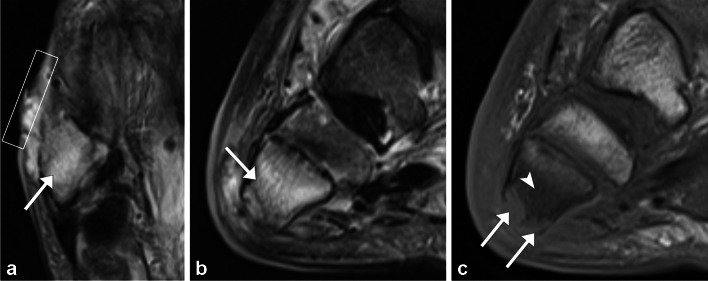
Seventy-one-year-old diabetic man with fifth ray infection status posttransmetatarsal amputation with positive margins. Long (a) and short (b) axis STIR images through the foot obtained several weeks after surgery demonstrate bone marrow edema like signal intensity approaching that of fluid within the fifth metatarsal stump (arrows). There is adjacent skin irregularity and abnormal T2 hyperintense subcutaneous signal (rectangle in a) without definite sinus tract. Short axis T1 MR image (c) shows cortical irregularity (arrows) and confluent medullary hypointense signal (arrowhead) within the fifth metatarsal stump. This patient underwent complete fifth metatarsal excision with pathology showing bony remodeling and chronic osteomyelitis with adjacent chronically inflamed soft tissue
